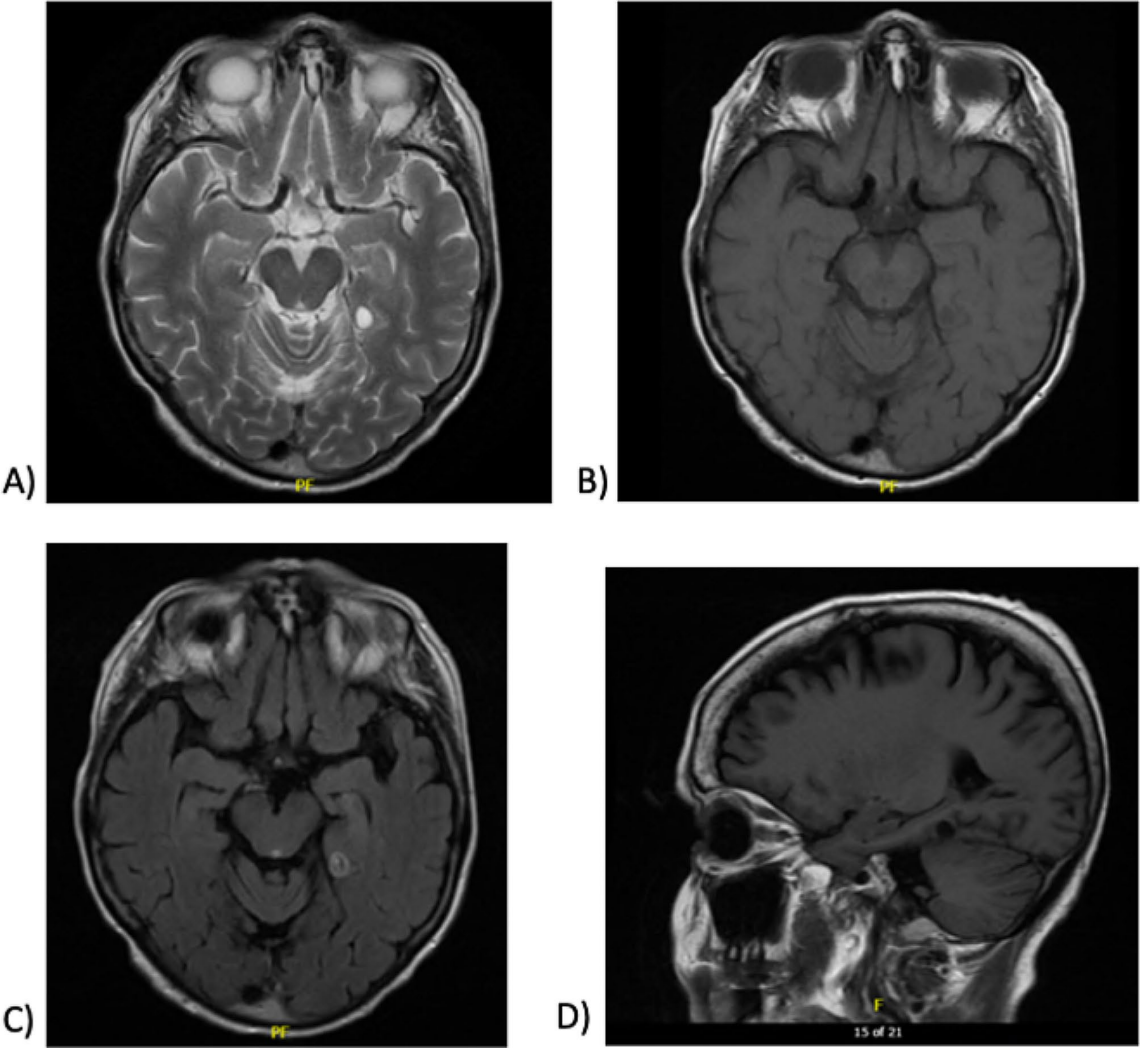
MRI Brain, November 2022, demonstrates the left ring enhancing posterior temporal lesion in the parahippocampal gyrus to medial occipitotemporal gyrus region, visible on imaging since September 2022. (A) Axial T2 Propellor. (B) Axial T1 Fast Spin Echo. (C) Axial T2 FLAIR post-gadolinium. (D) Sagittal T1 FLAIR
